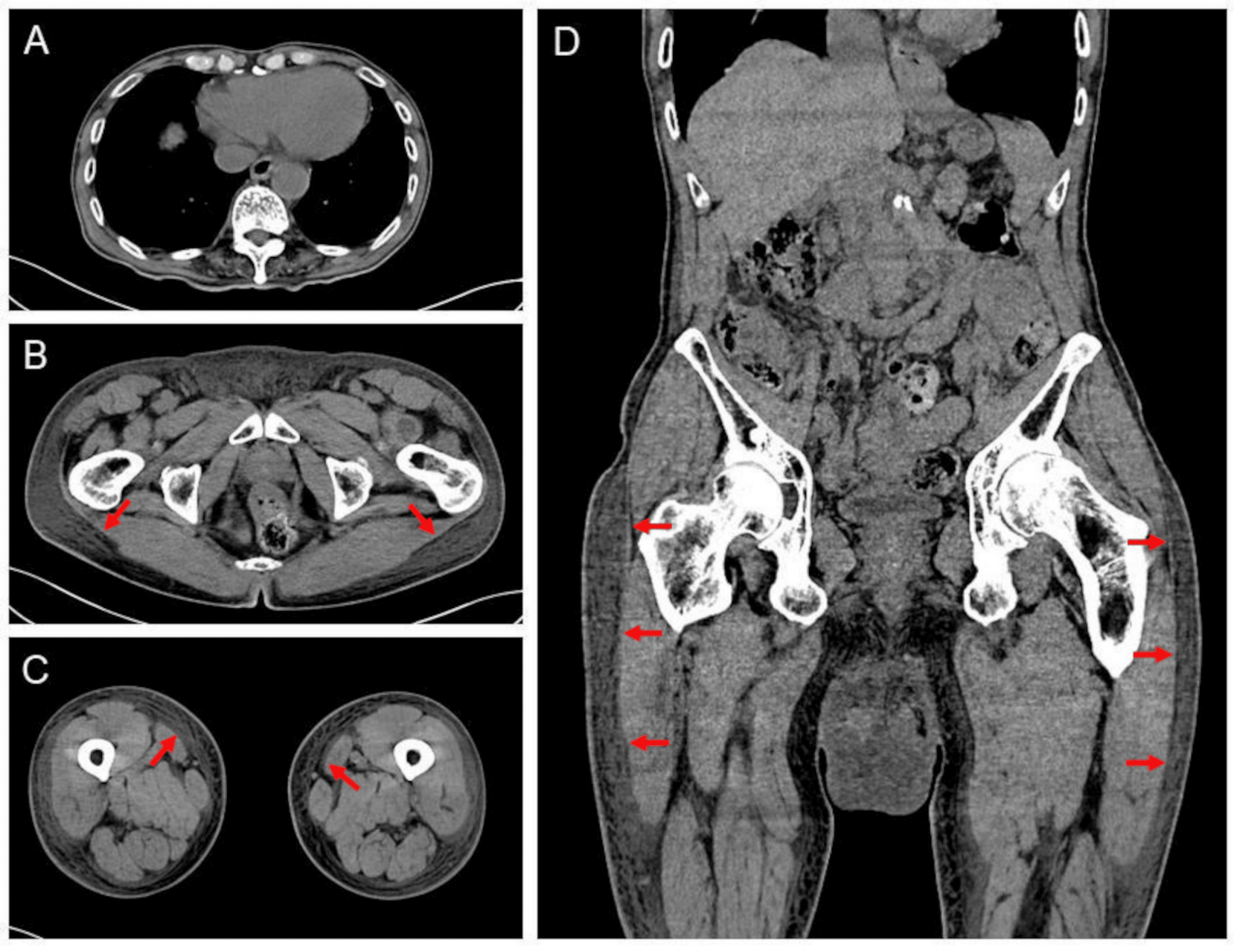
Computed tomographic images revealed no pleural effusion in the thorax (A). There were no significant abnormalities, such as malignancies inhibiting venous return in the abdomen. Excessive edema was distributed below the waist (B-D, red arrows)
